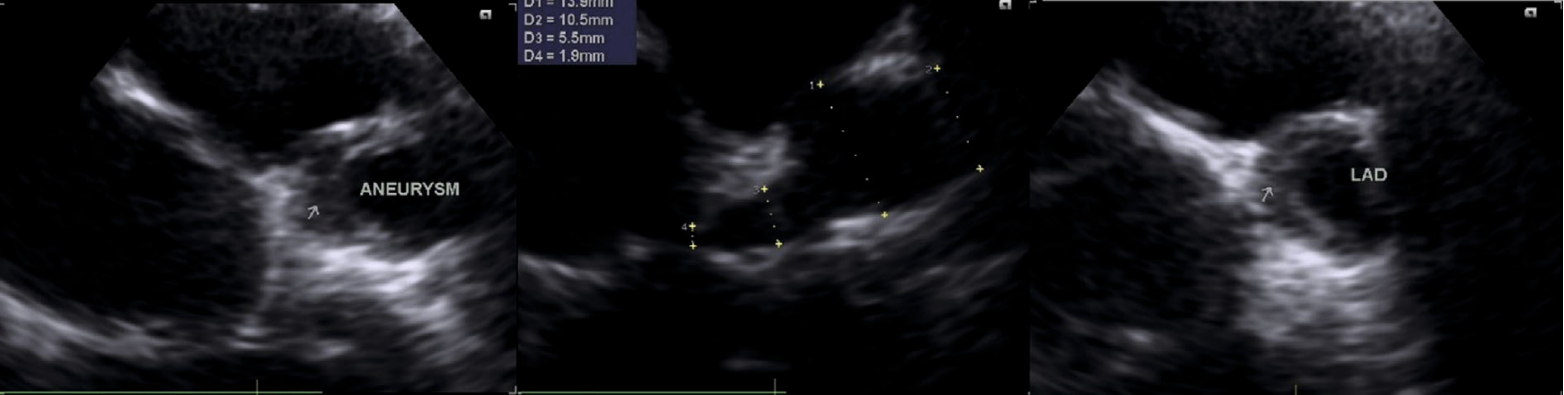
Short axis view (SAX) of transthoracic echocardiography (TTE) in our patient shows ectasia at left anterior descending (LAD) artery and huge aneurysm with large circular nonorganized thrombosis occupying the majority of the aneurysm, narrowing the internal lumen of the artery.
